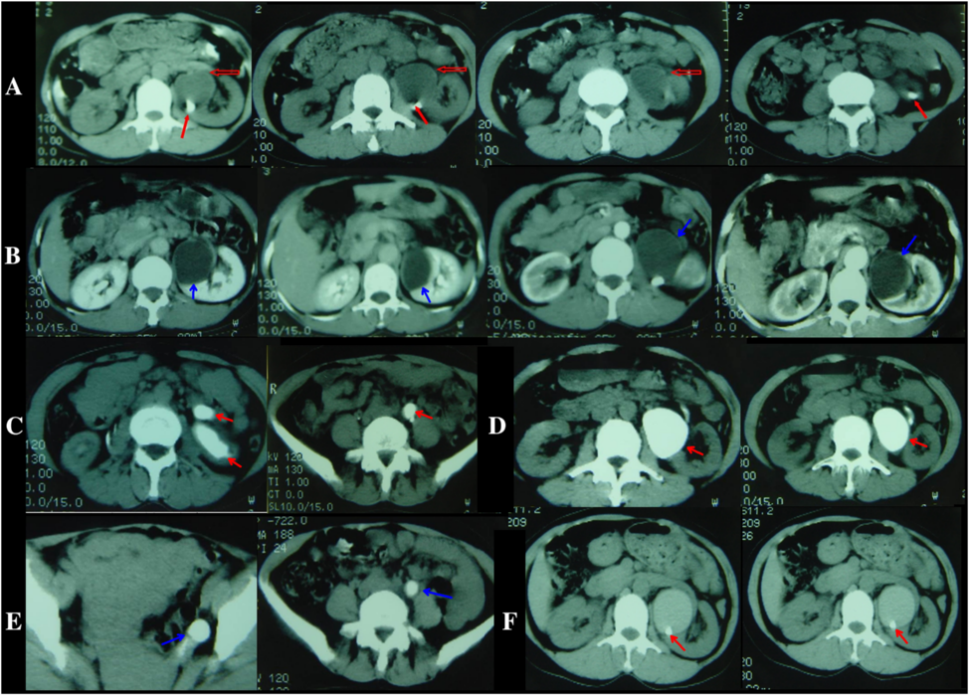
Case 2. CT scans obtained in a 46-year-old woman with left duplex kidney & ectopic ureter openings. Non- enhanced scan obtained at the level of the upper-middle pole of the left kidney shows areas of abnormal low-density (*red faint arrow*) and stone shadow (*red solid arrow*) (a). Contrast-enhanced scans obtained at the same level as in (a). The upper-middle pole of the left kidney (blue arrow) shows no enhancement on the 6-min scan (b) and abnormal low density enhancement on the 3 h (c), 18 h (d), 24 h (e) and 45 h (f) scan, and ectopic ureter openings can be seen in (e) (blue arrow)
